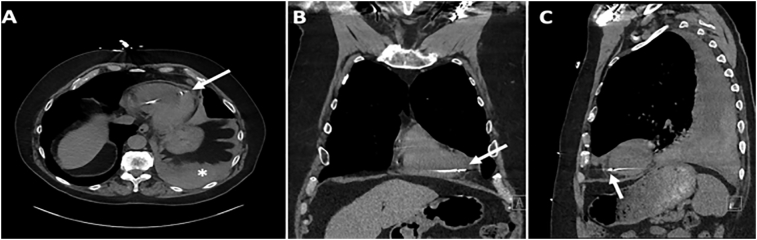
Chest computed tomography in the axial (left panel; A), sagittal (middle panel; B), and coronal (right panel; C) planes showing RV pacing lead, intact pericardium, and no effusion; the asterisk (*) denotes the pleural effusion.
